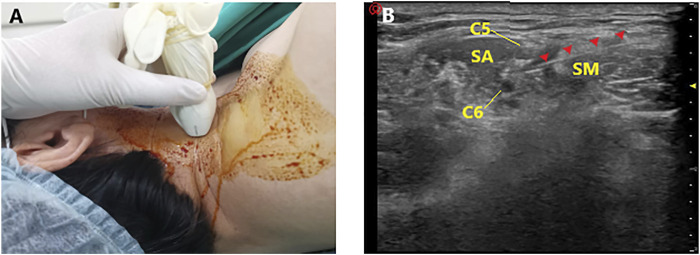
Ultrasound-guided interscalene brachial plexus block. (A) Placement of the ultrasound probe. (B) Ultrasonography of interscalene brachial plexus block (SA, scalenus anterior; SM, scalenus medius; Red arrow: ultrasound image of the needle).
